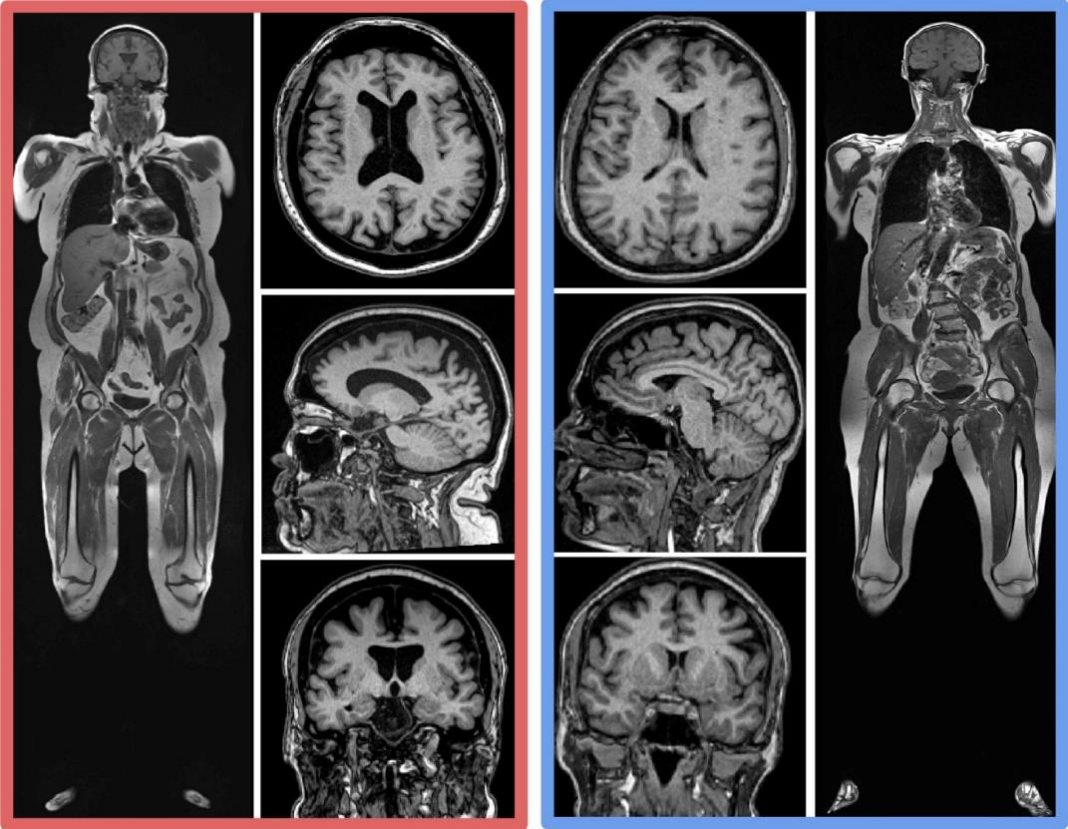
Examples of Higher Body Fat and Brain Volume Loss. This figure shows two examples of coronal T1 weighted whole body MRI in 61-year-old women, one with a high level of both vfat and sfat (red border) and the other with a normal amount of vfat and sfat (blue border). There is corresponding increased brain atrophy in the high vfat and sfat person as evidenced by enlarged ventricles and widened sulci on the T1 brain MRI images.
